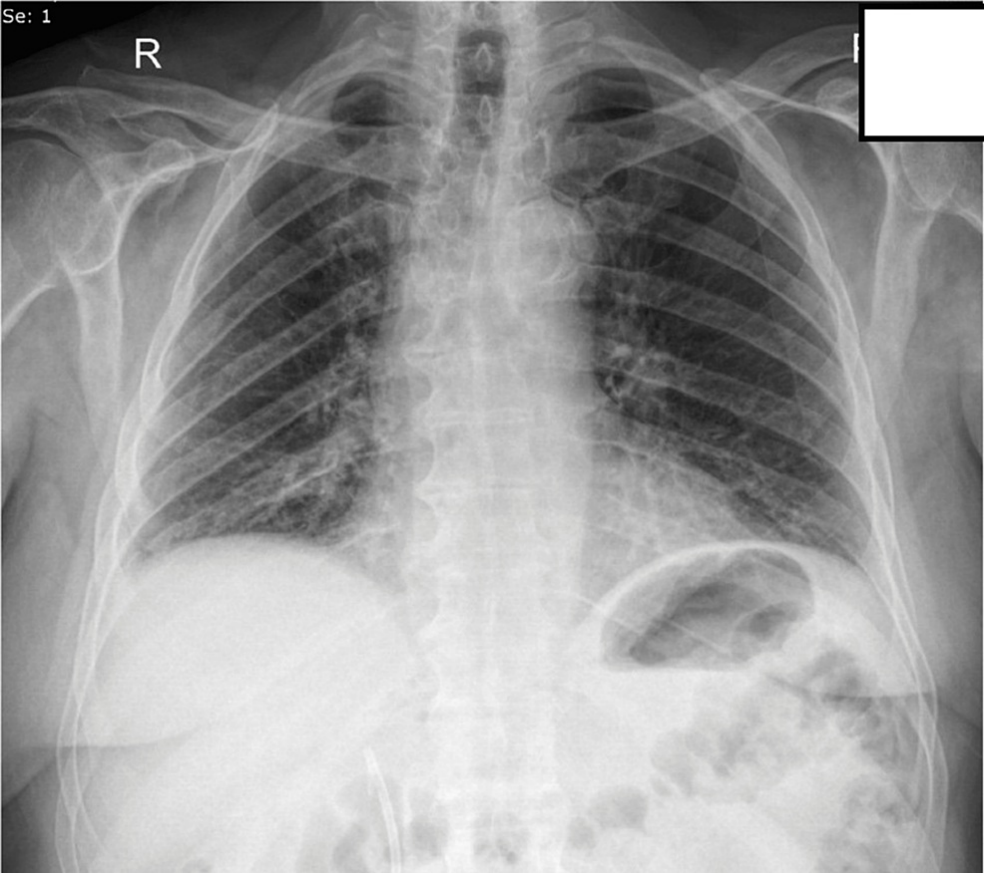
Chest X-ray one month post-discharge No pathological radiographic findings were noted.
